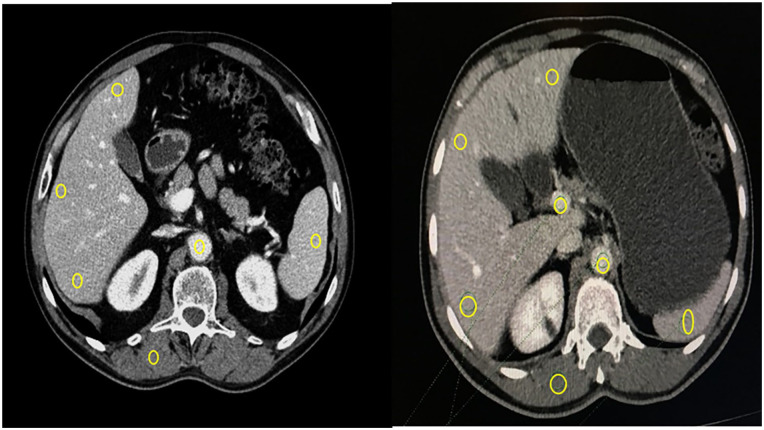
ROI placement in different organs. Figure adapted from “Optimising contrast volume based on body mass index in low tube voltage CT of abdomen and pelvis” by Panakkal N C. EPOS (online) ECR2025.10.26044/ecr2025/C-15098. https://epos.myesr.org/poster/esr/ecr2025/C-15098
